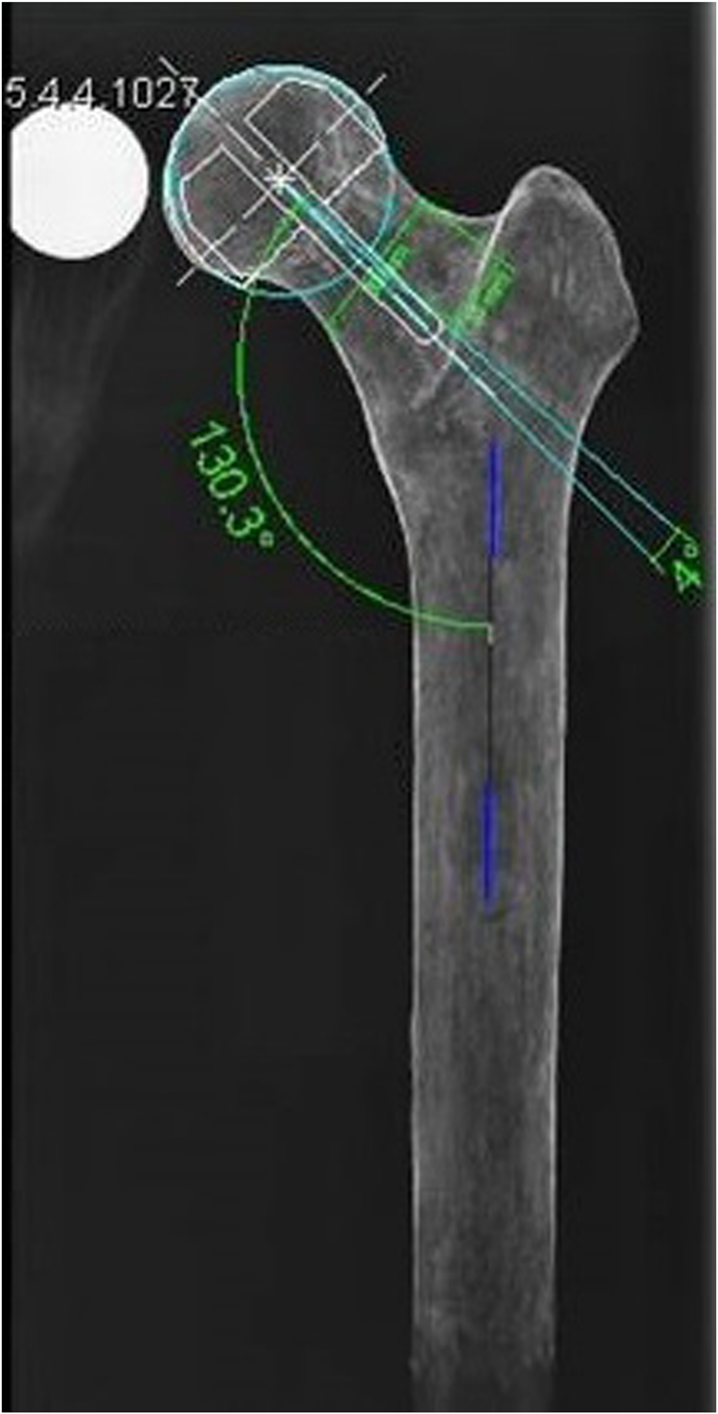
Representative X-ray image including CCD angle (here 130.3°), planned position (here stem shaft angle 134°), and size (here “44”) of the femoral component.
